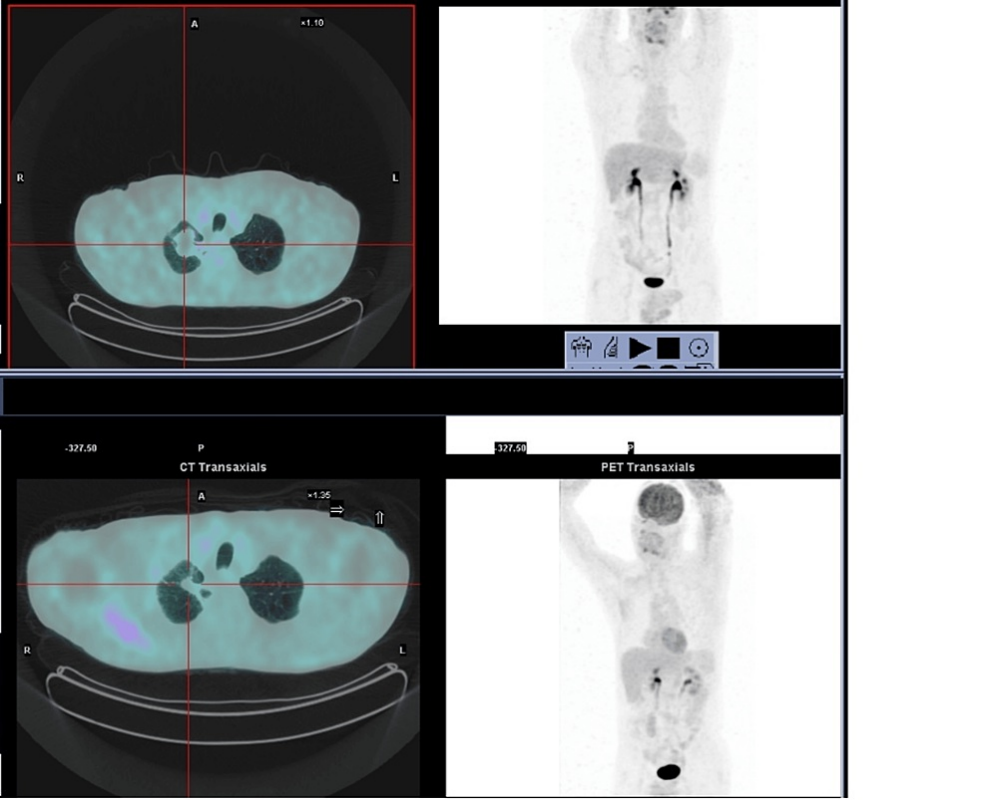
FDG/PET-CT after pembrolizumab and SBRT to the lung mass in April 2022
